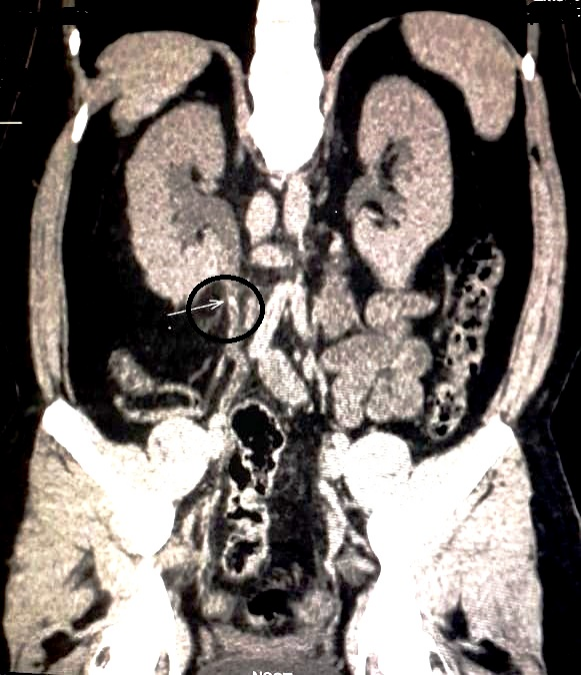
Computed tomography (CT) of the abdomen showing a mass lesion in the right renal pelvis
